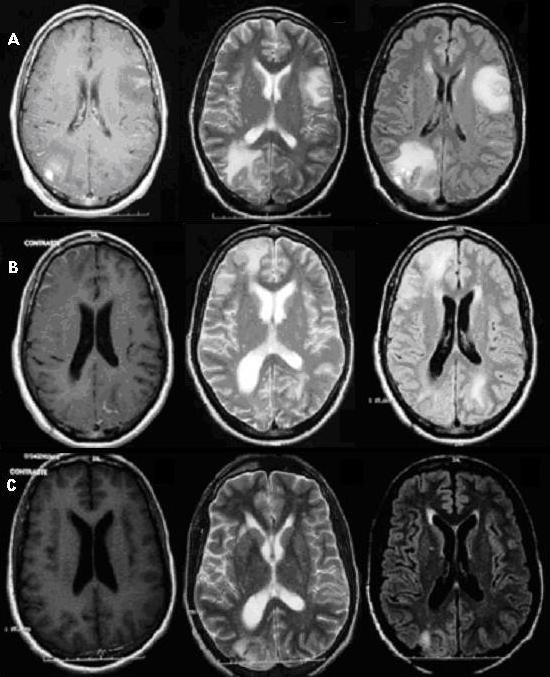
Panel A. Intraoperative brain images in April 2004. An axial T1-weighted image after gadolinium injection (left-hand side) shows lesions in the gray matter of the left temporo-parietal lobe and right occipital lobe (place of biopsy). These images show slight enhancement of the lesions particularly in the meninges and the presence of mass effect. An axial T2-weighted image (center) and a FLAIR image (right-hand side) show better details of the lesions. Panel B. Brain MRI images, 5 months after discharge. Axial T-1 weighted image after gadolinium injection (left-hand side) showed important improvement in gray matter lesions. However, images in T2-weighted (center), and FLAIR (right-hand side) showed presence of new high-signal-intensity lesions in the white matter of the right frontal lobe and left occipital lobe. A widening of the right ventricle compared to the figure in Panel A can be observed. Panel C. Brain MRI images, 7 months after discharge. Axial T-1 weighted image after gadolinium injection (left-hand side) shows normal appearance. Images in T2-weighted (center) and FLAIR (right-hand side) reveal regression of the white matter changes; however discrete widening of the right ventricle is still present.
